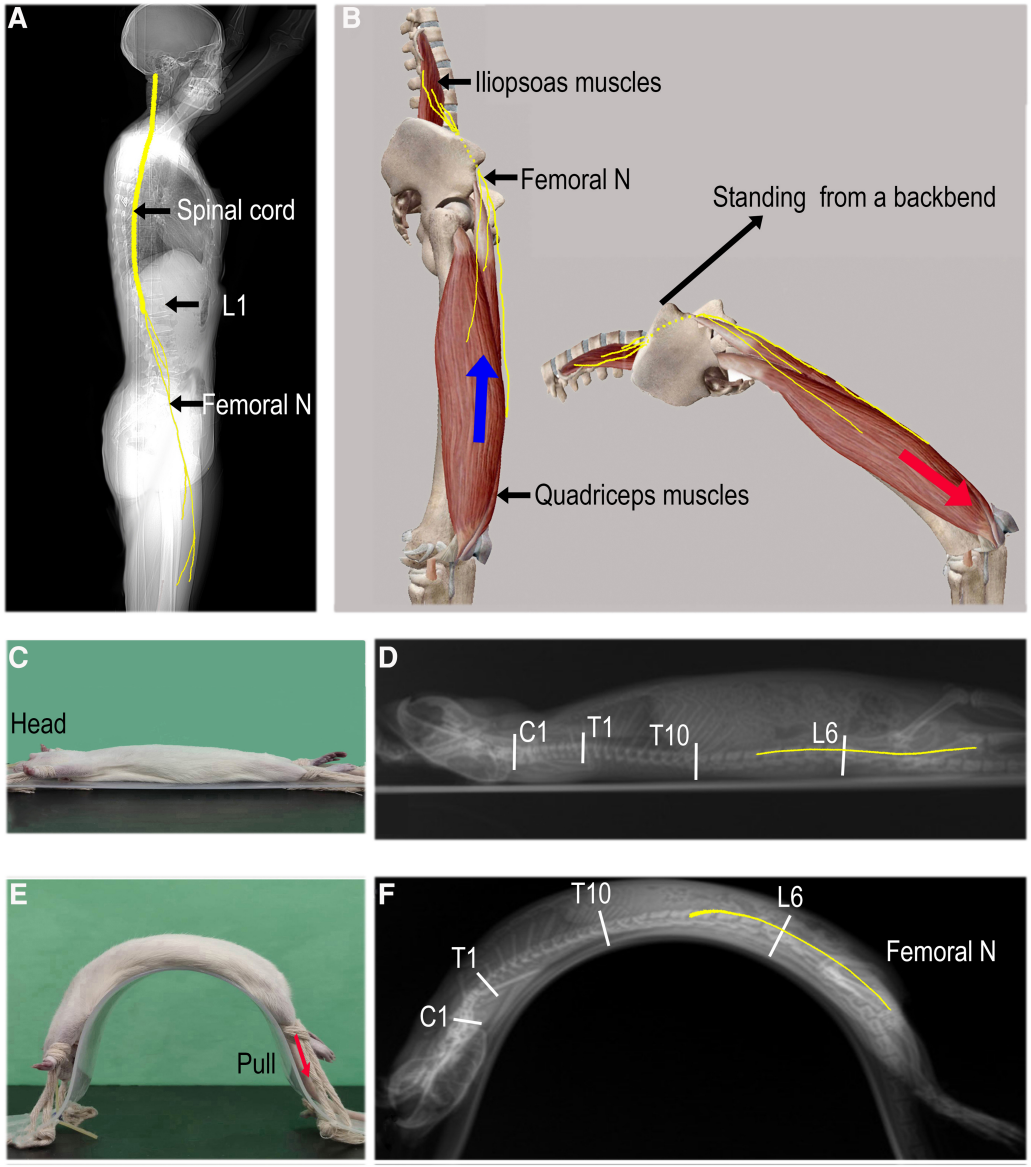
Mechanism of femoral nerve overstretching. (A) The spinal cord (C1–L1) and femoral nerves (originating from L2–L4 nerve roots). (B) The femoral nerves are overstretched from a standing position to a full backbend and are further pulled distally if a sudden eccentric contraction of the quadriceps occurs (red arrow). (C and E) In the supine and passive full backbend positions, respectively. The unilateral low limb is rapidly pulled distally to stretch the femoral nerves (red arrow); (D and F) a lateral x-ray of the whole spine with estimated lengths of the spine and femoral nerves (yellow lines).
